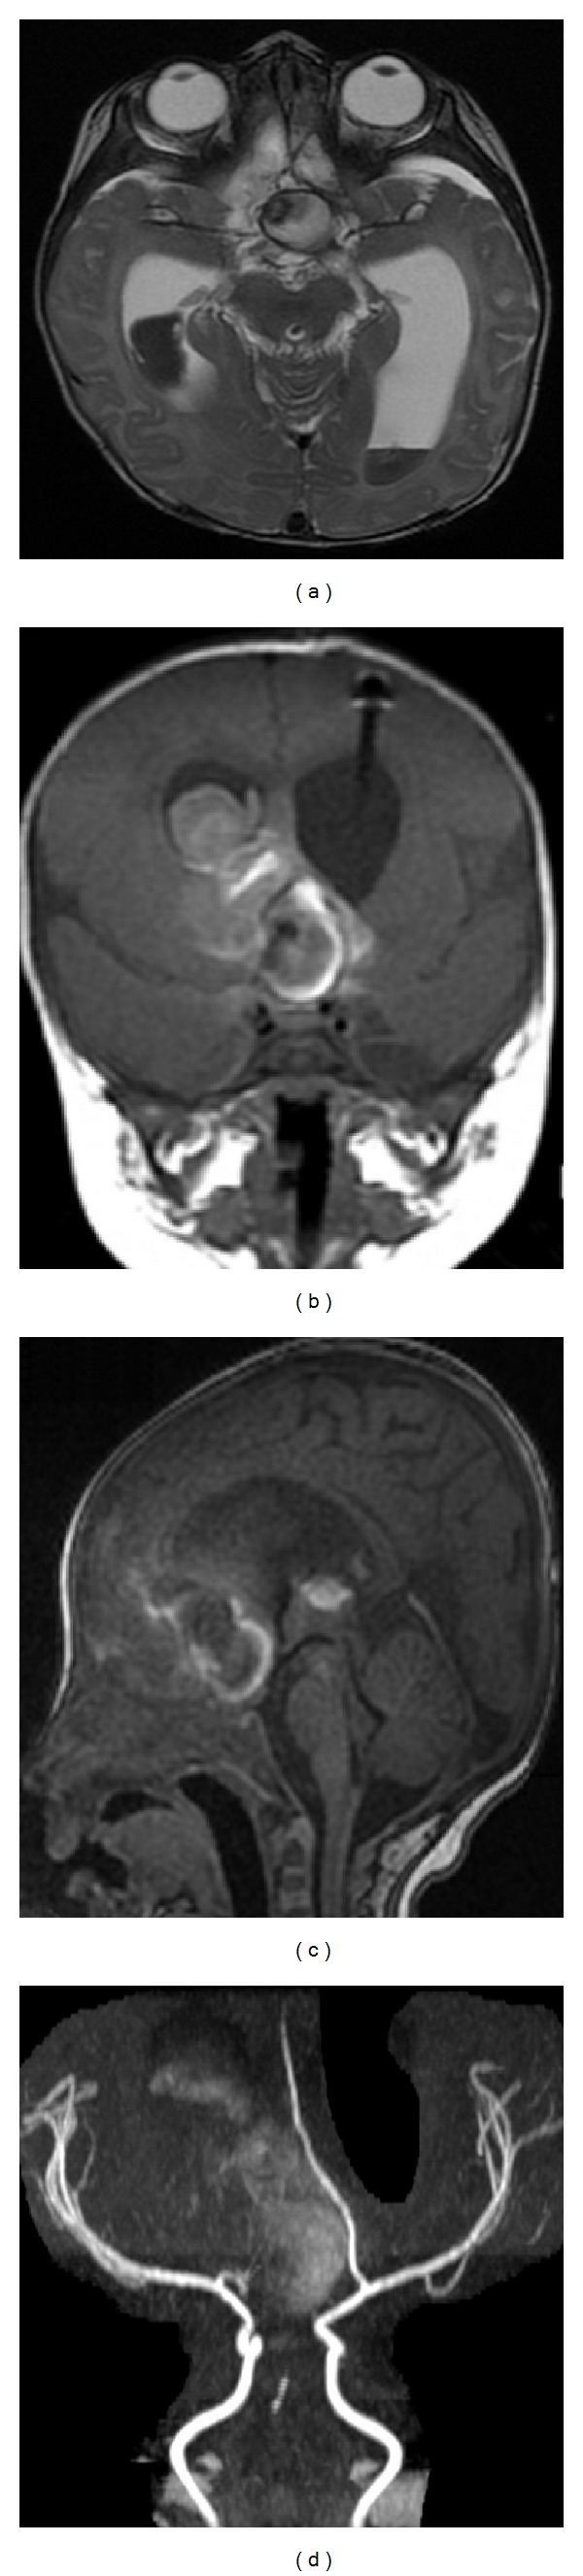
((a), (b), and (c)) Early stage MRI demonstrating intraventricular hemorrhage with associated hydrocephalus and a large extra-axial suprasellar lesion measuring 26 mm diameter with a slight filling and an endoluminal thrombus; (d) on MRA no lesion is visualized, likely due to its partial thrombosis and sluggish blood flow. Only its repercussion on middle caliber vessels can be appreciated: left A1 segment of the anterior cerebral artery superiorly and laterally deviated and right A1 only visible on its proximal segment.
